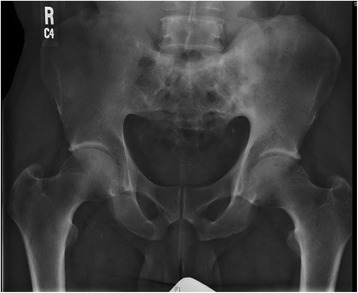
Pelvic X-ray on presentation. The patient’s pelvic film demonstrates thickened trabeculae and sclerosis in addition to bony ankylosis of sacroiliac joints bilaterally. The trabecular pattern appears to partially extend into the *left medial iliac region*
